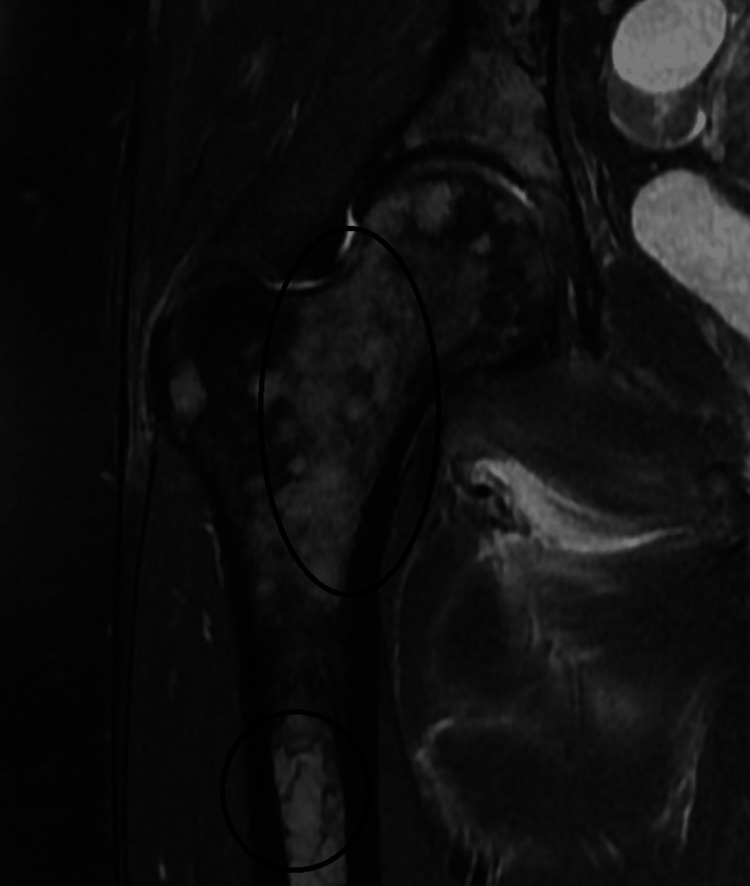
T2-weighted coronal magnetic resonance imaging of the right proximal femur. *Black circles*: Extensive marrow replacement process visualized in the proximal femur.
